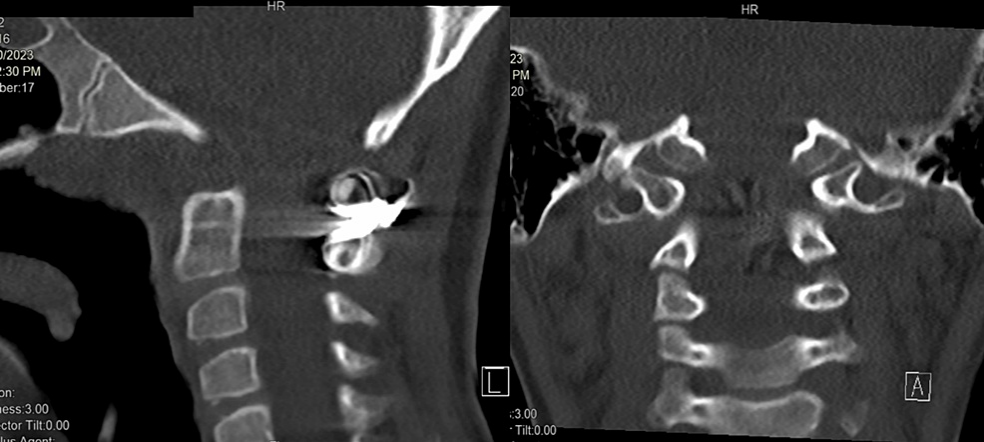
CT scan sagittal and coronal image at three months post-surgery shows a widening of the spinal canal CT: computed tomography
